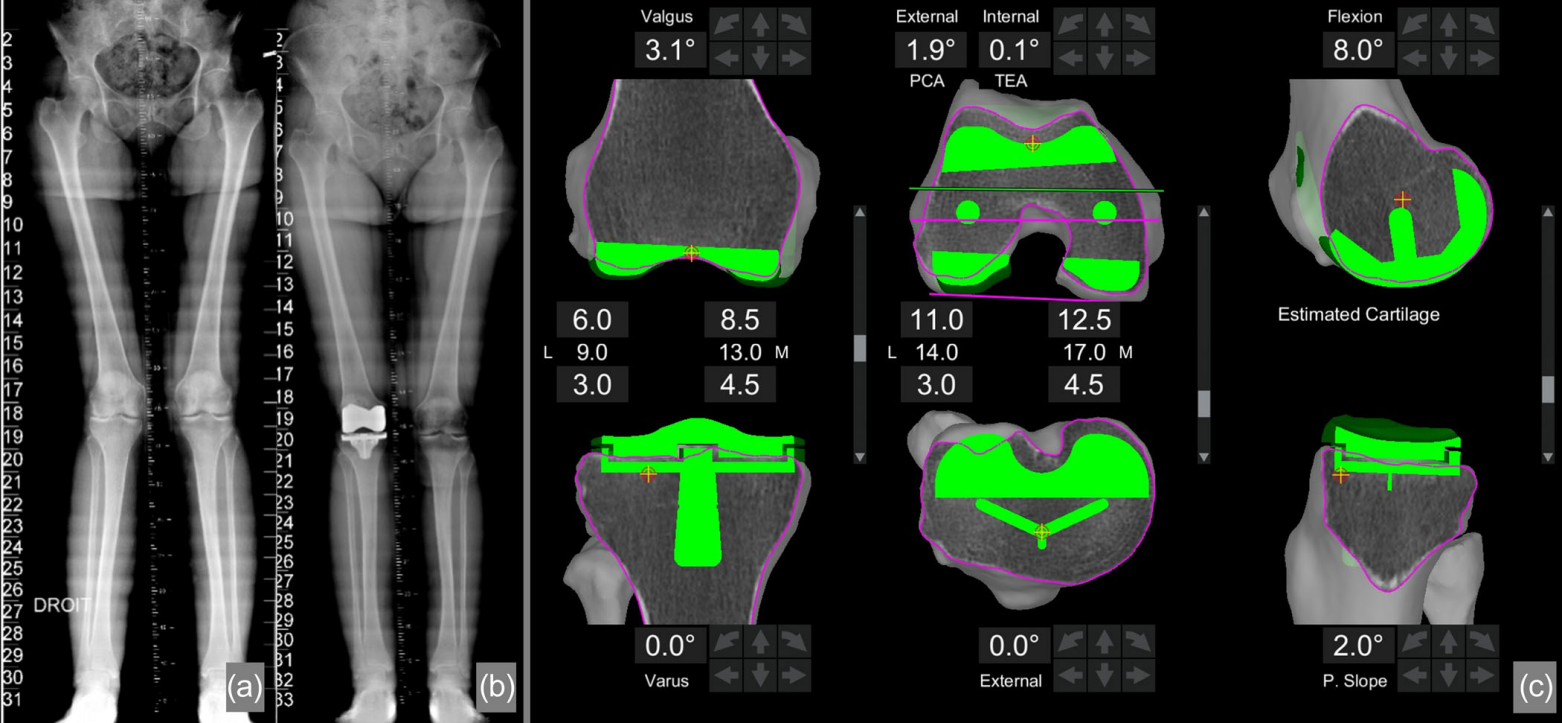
Seventy‐two‐year‐old female patient with a major valgus coronal deformity, presenting a preoperative mechanical hip–knee–ankle (mHKA) angle of 188° on the right side, corrected to 187° after total knee arthroplasty (TKA). (a) Preoperative long‐leg standing radiograph; (b) postoperative long‐leg standing radiograph; (c) intraoperative screenshot of TKA performed with the Mako® robotic system (Stryker ®), showing planning adjustments according to functional knee positioning principles.
